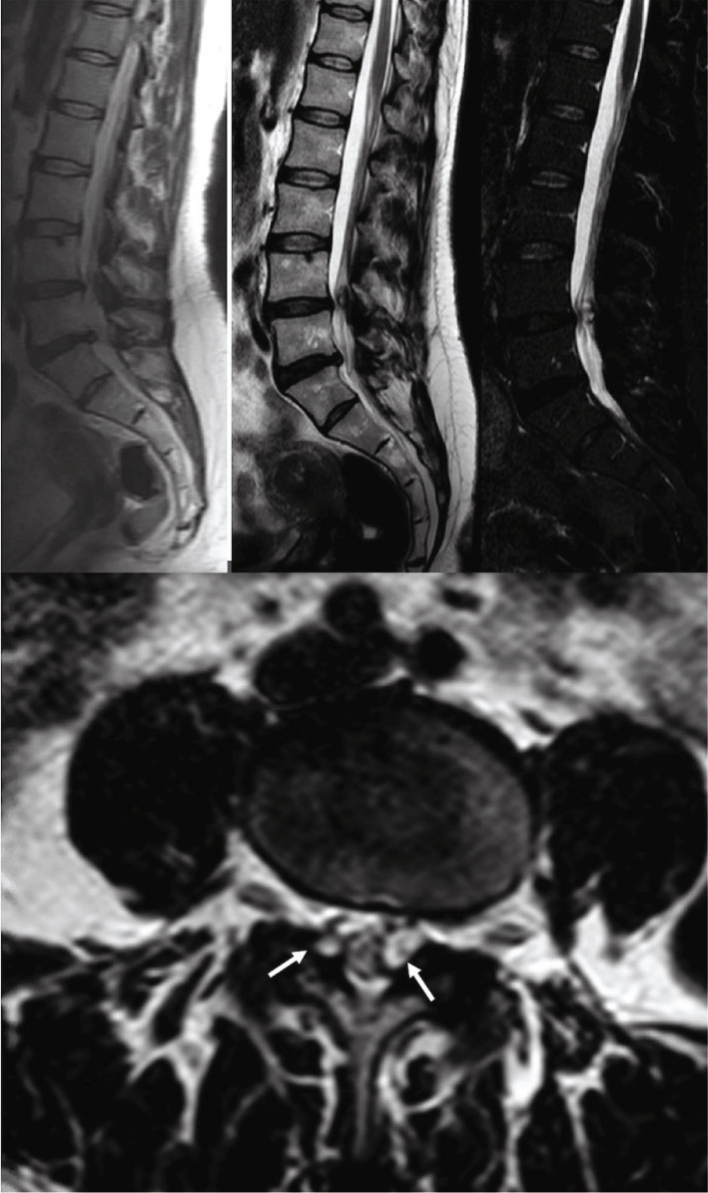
MR images on T1, T2, and STIR sequences. Hypointensity on T1 sequences and hyperintensity on T2 sequences. In sagittal cuts, we can see the cyst and how it compromises the spinal canal, as well as degenerative changes in L4-L5/L5-S1. In axial cuts, L4-L5 bilateral cysts can be seen (white arrows).
